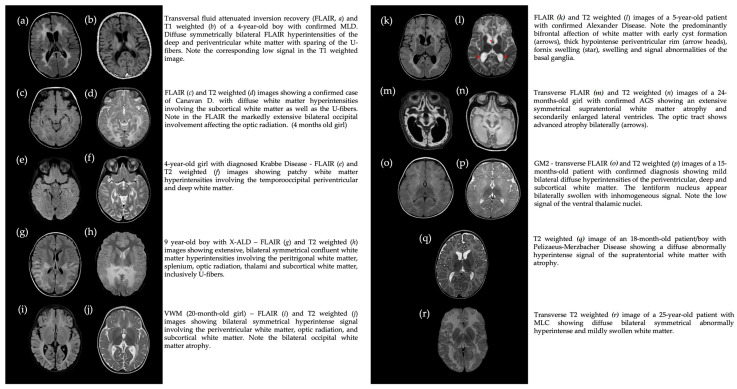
Pathological brain MRI findings of selected patients with myelin involvement of the visual pathway.
